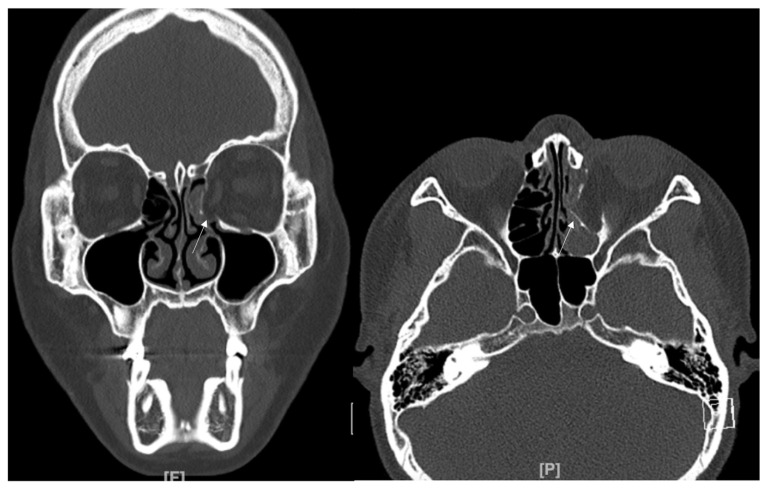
Case 3. Preoperative facial CT scan (coronal and axial view) of the 34-year-old female patient with left medial orbital wall fracture (white arrow). The fracture sites were designated with white arrows.
